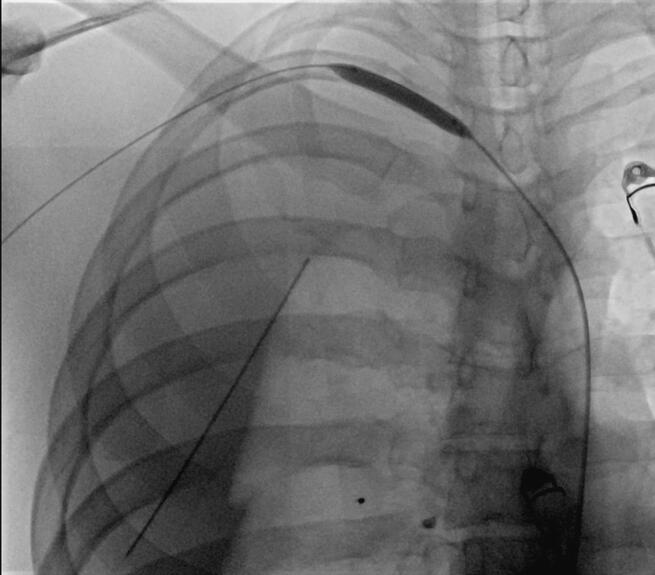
An X-ray shows the use of an undersized balloon used to control extravasation.
